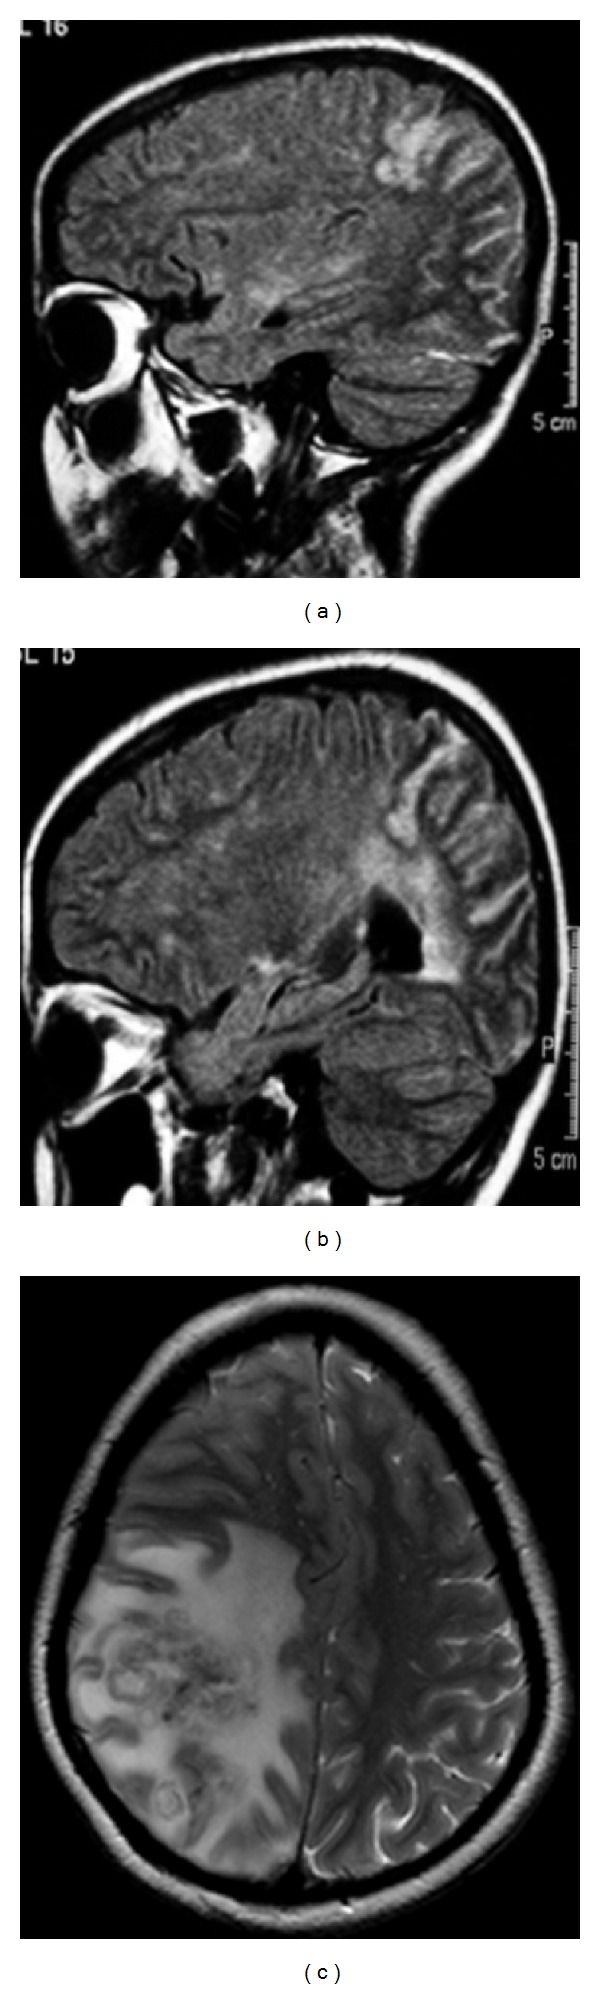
(a) MRI brain 5 days into admission in August 2005 showing right-sided occipitoparietal leptomeningeal inflammation. (b) MRI brain on readmission December 2005, 4 months after initial presentation showing worsening right-sided occipitoparietal leptomeningeal inflammation. (c) T2-weighted MRI brain in December 2006, 16 months after initial presentation showing large clusters of ring-enhancing lesions and leptomeningeal inflammation in the right occipitoparietal area with oedema and midline shift. Also seen but not shown on this cross-section were smaller clustered ring-enhancing lesions in the right thalamus and left frontal and temporal lobes.
